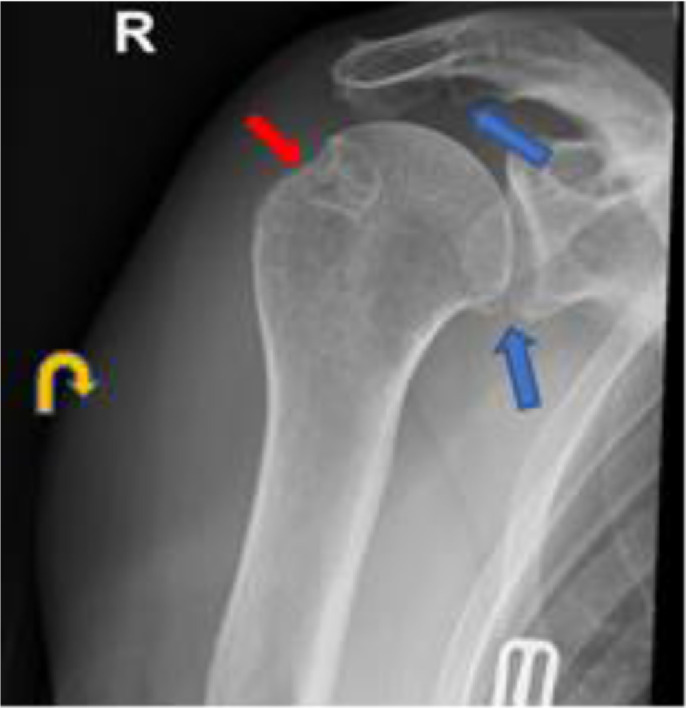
Frontal radiograph of the right shoulder demonstrates osteoarthritis of the acromioclavicular and glenohumeral joints with small marginal osteophytes (blue arrows). There is subacromial spurring and post-surgical changes of the greater tuberosity following prior rotator cuff repair (red arrows). There is a noncalcified soft tissue mass along the lateral aspect of the shoulder (curved yellow arrows), without evidence of underlying periosteal reaction, erosion, lytic or blastic osseous lesion.
